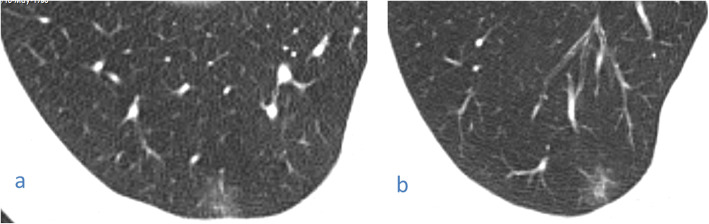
A 60-year-old male patient with COVID-19, CT chest axial image (a) shows subpleural ground glass opacity with vascular thickening and (b) shows a subpleural nodule with “halo” sign
